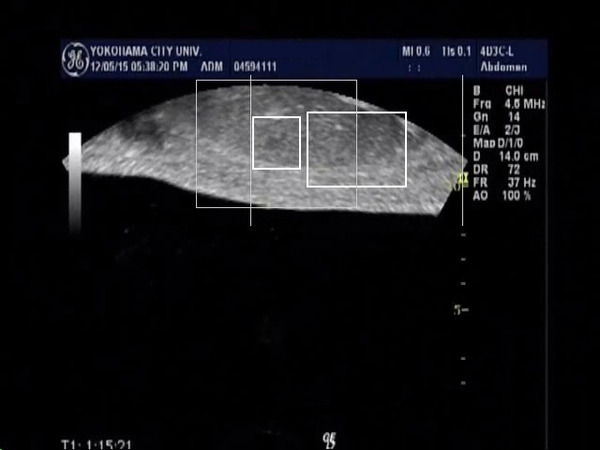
Tumor of the monitor ultrasonography was tracked in the small square, and it was tracked successfully in breath out position
